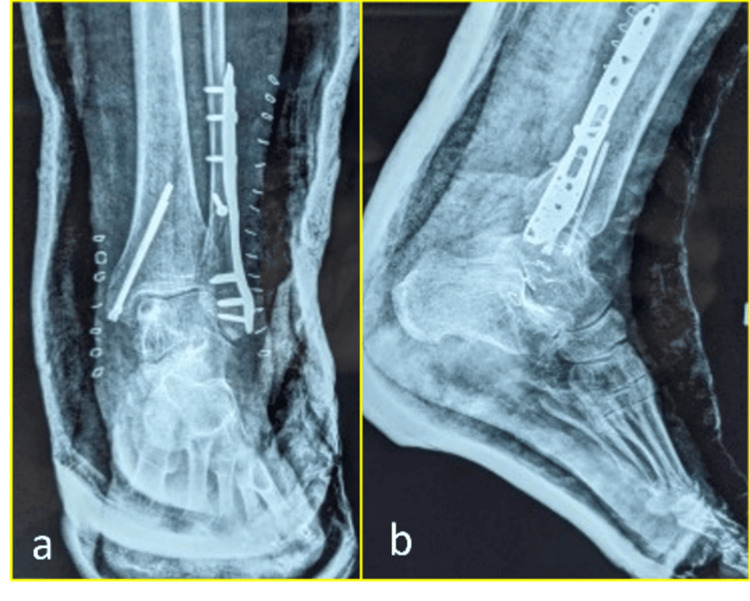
Postoperative anteroposterior and lateral radiographs confirming anatomical reduction and screw placement in a bimalleolar fracture: (a) anteroposterior view and (b) lateral view The lateral plate for fibular fixation and headless screw for the medial malleolus are visible.
